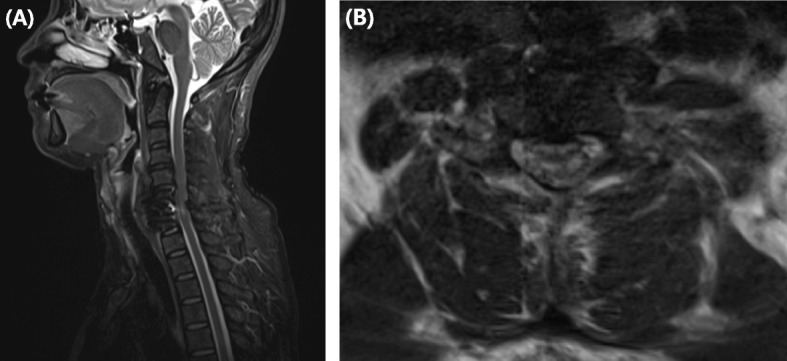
a Immediate postoperative sagittal STIR (Short tau inversion recovery) MR image shows cervical anterior decompression state and high signal intensity at C6–7 level. b Immediate postoperative axial T2 weighted MR image shows anterior bilateral symmetric ovoid foci of high signal intensity at C6–7 level
